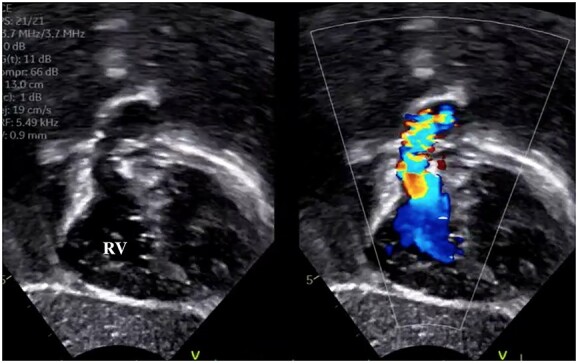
Preoperative transthoracic echocardiography showing right ventricular outflow tract narrowing and jet flow.
